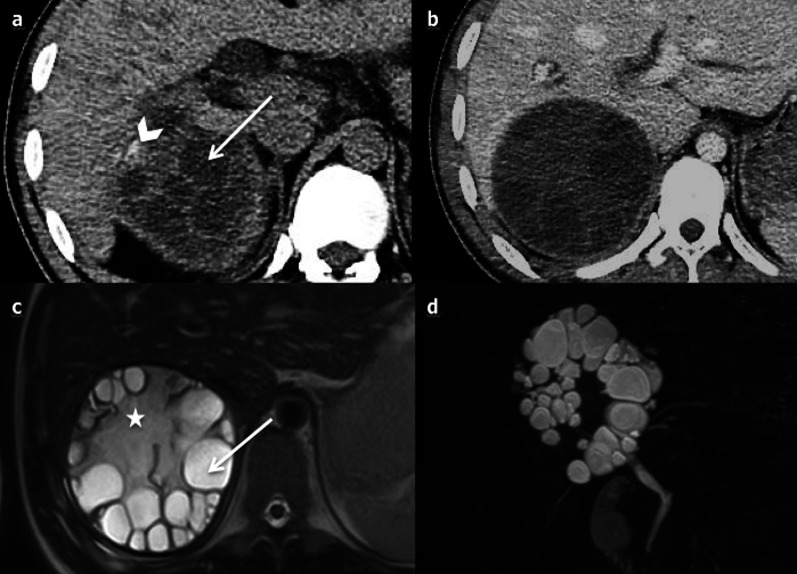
cyst echinococcosis 2, in a 50-year-old male. a Axial non-enhanced computed tomography shows a heterogeneous mass with a cystic part (arrow) and calcification of the wall (arrowhead). b On axial computed tomography on portal venous phase no enhancement of the cystic component is observed. c The cystic part and the differentiation between the daughter (arrow) and the mother (star) cysts are better evaluated on axial T2-weighted magnetic resonance imaging. d Coronal 3D magnetic resonance cholangiopancreatography excludes biliary fistula from the possible diagnosis
